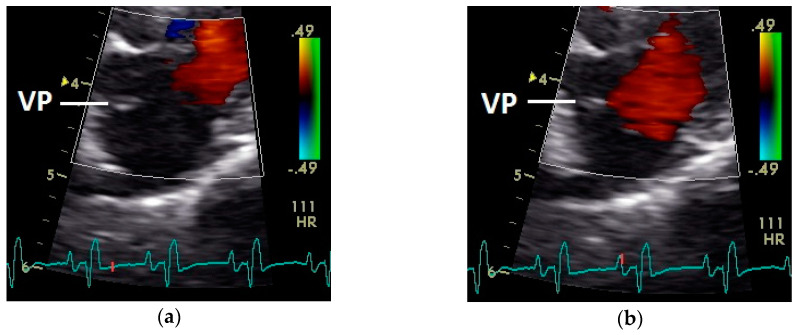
Position of the valva pulmonis (VP) in the diastole: (a) passive and (b) active ventricular filling (red signal); HR: heart rate; color scale of the color Doppler image is calibrated in m s^−1^.
